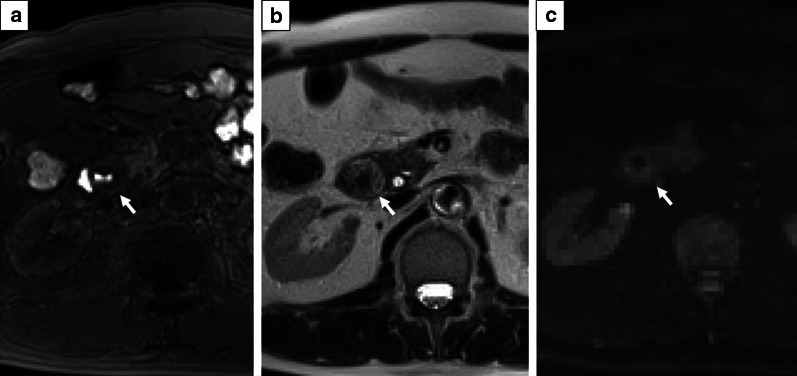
a The tumor revealed hypo-intensity on T1-weighted image. b The tumor shows a modestly high intensity on T2-weighted imaging. c The tumor shows a slightly high intensity on diffusion-weighted images
